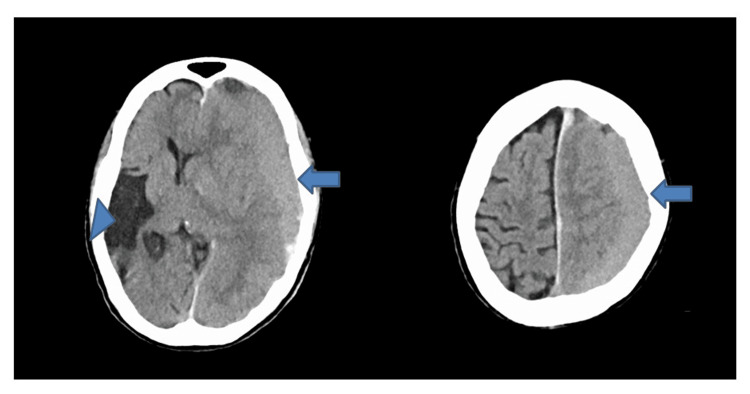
An initial head CT obtained 10 minutes after arrival showed a left chronic subdural hematoma (20 mm thick) (arrow) with a 15 mm midline shift to the right and a hypodense area occupying the right temporoparietal region (arrowhead), with no contralateral hematoma.
